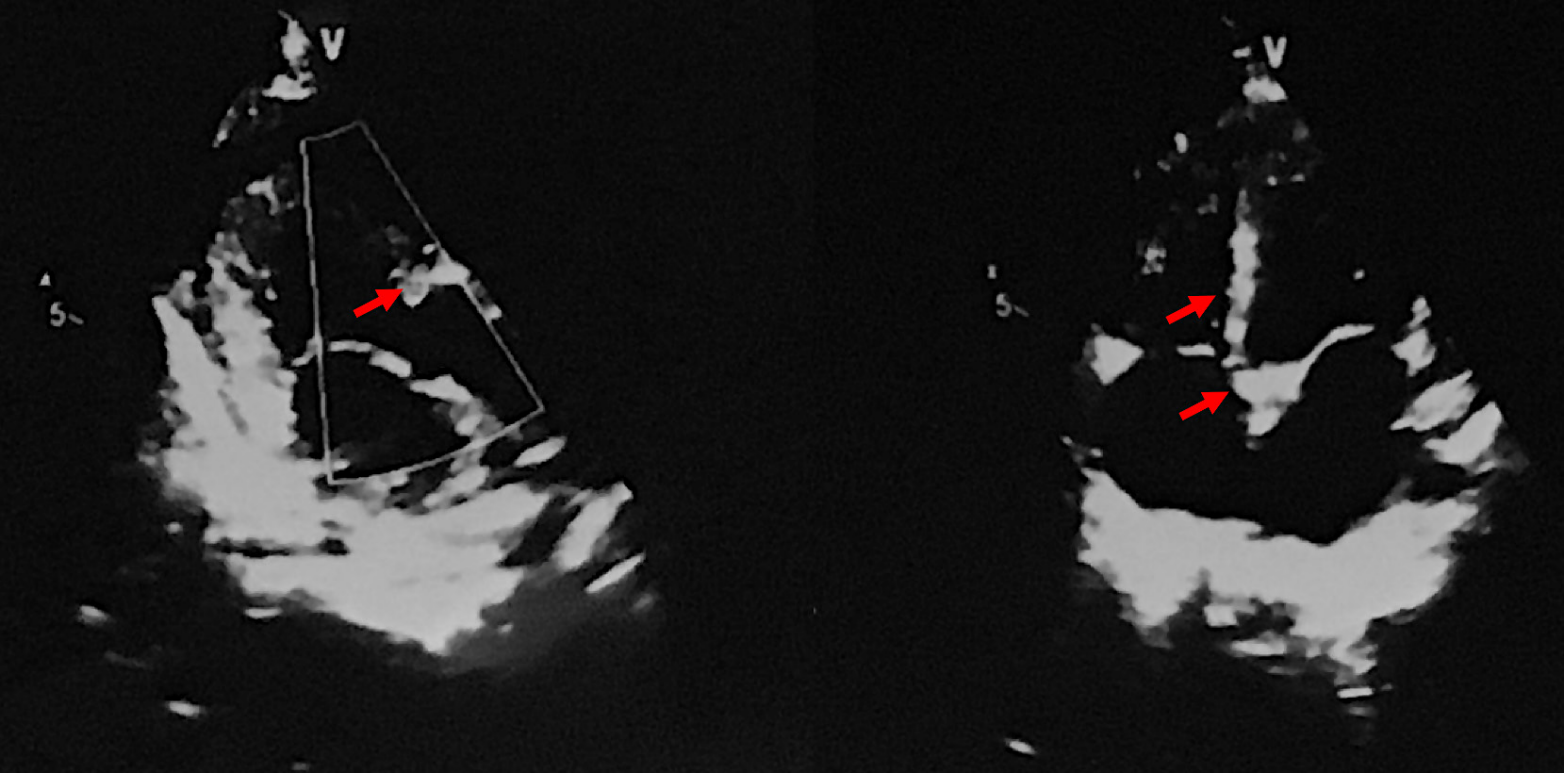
Echocardiogram revealed the rhabdomyoma that compromised interventricular septum, left ventricle and aortic valves (red arrow).
